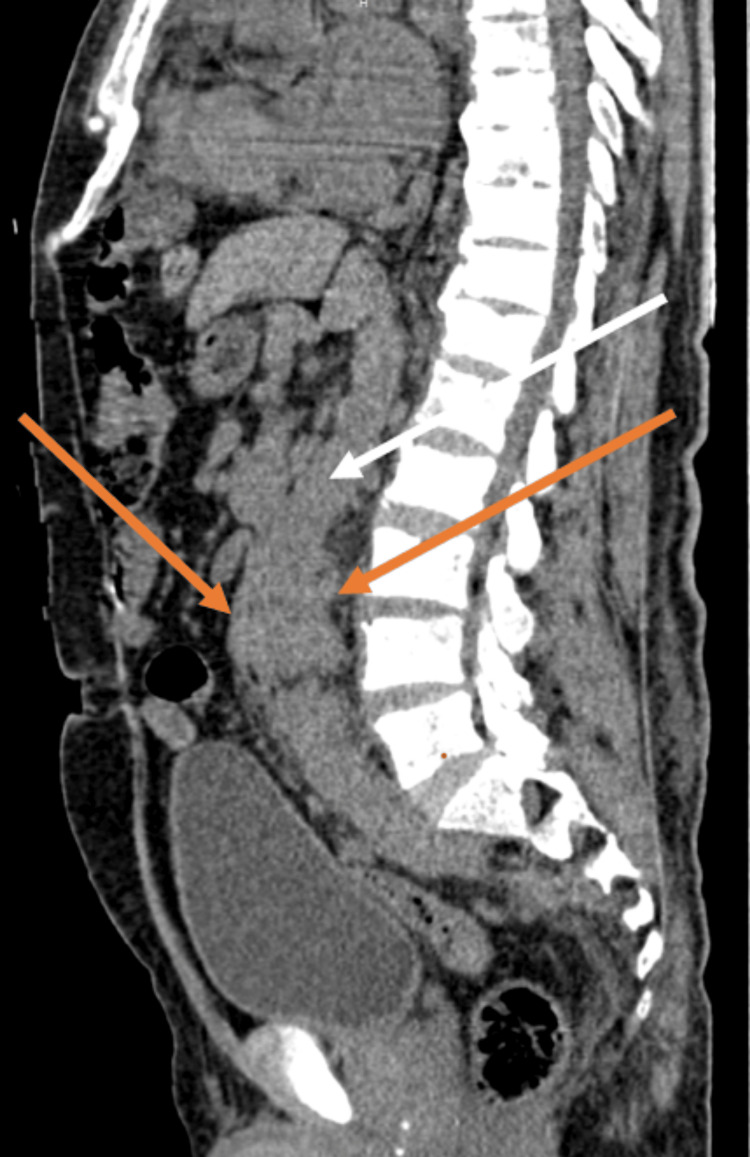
Sagittal section of computed tomography of kidney, ureters and bladder showing hypodense mass lesion circumferentially encasing the descending aorta (Orange arrows) and white arrow indicates the descending aorta.
